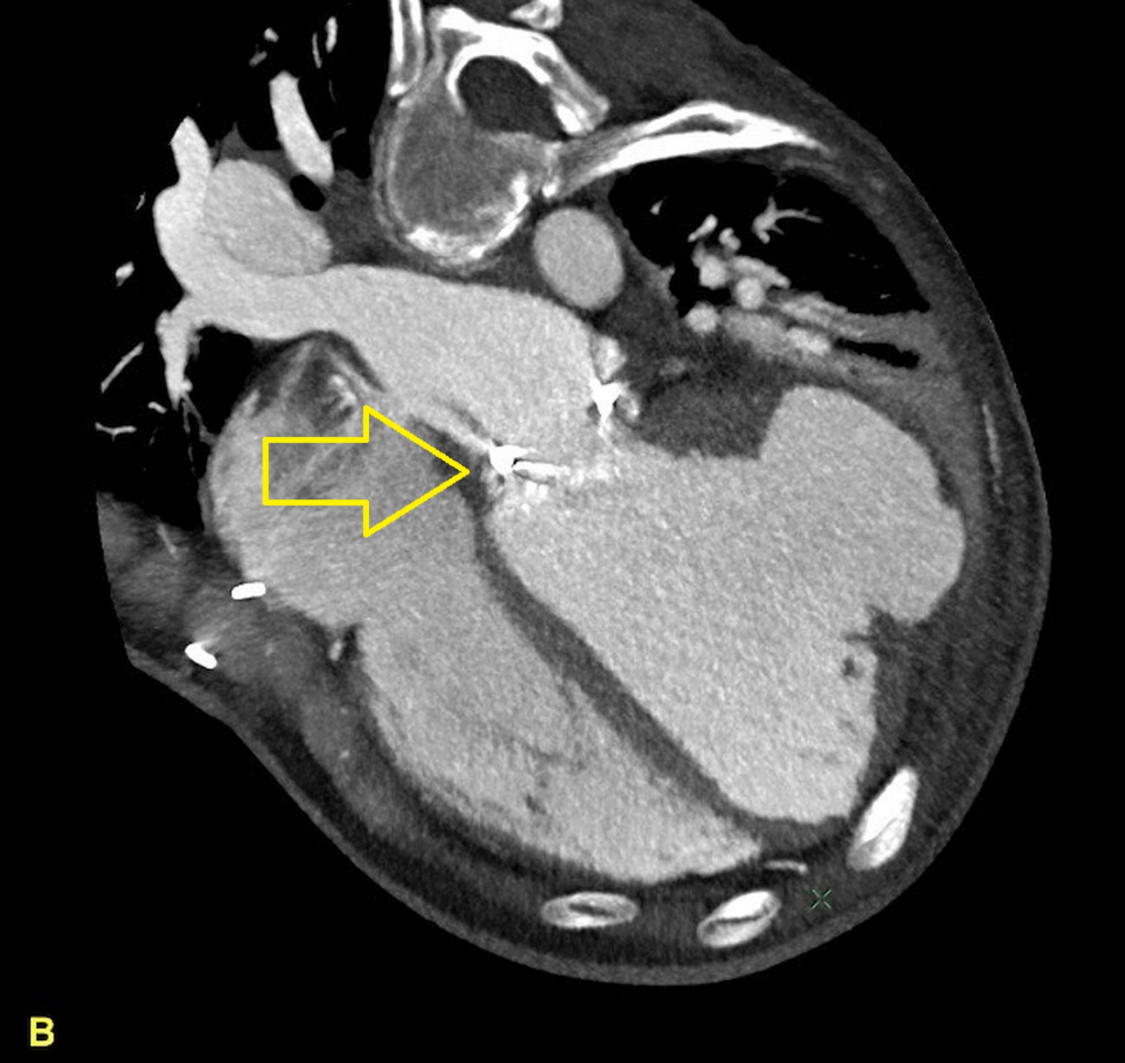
Cardiac computed tomography image showing other cardiac chambers along with the pseudoaneurysm (PA).
